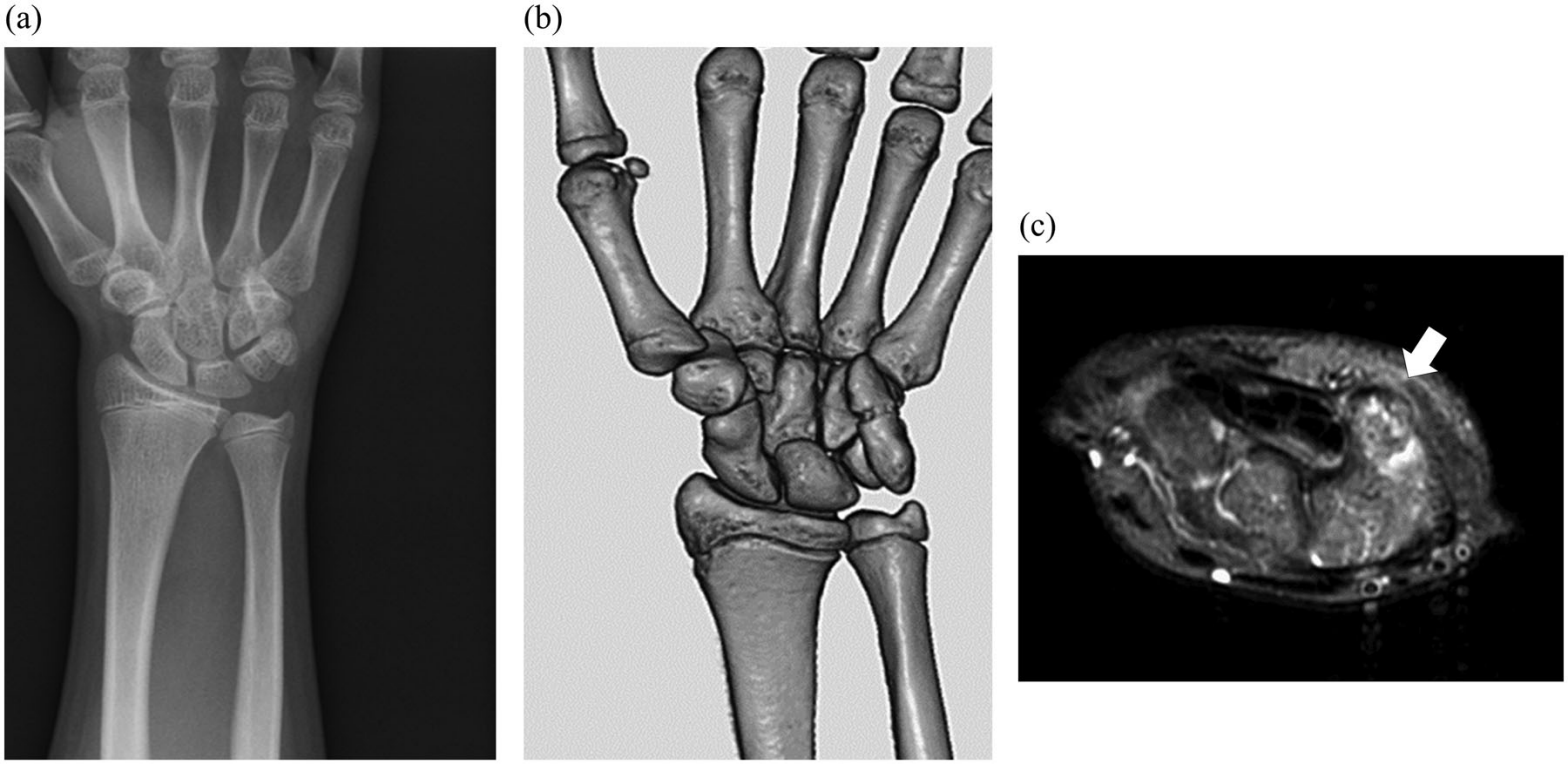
(a) Plane X-ray before the surgery (b) 3D reconstruction CT scan before the surgery (c) T2 image of MRI; white arrow indicates pisiform.
